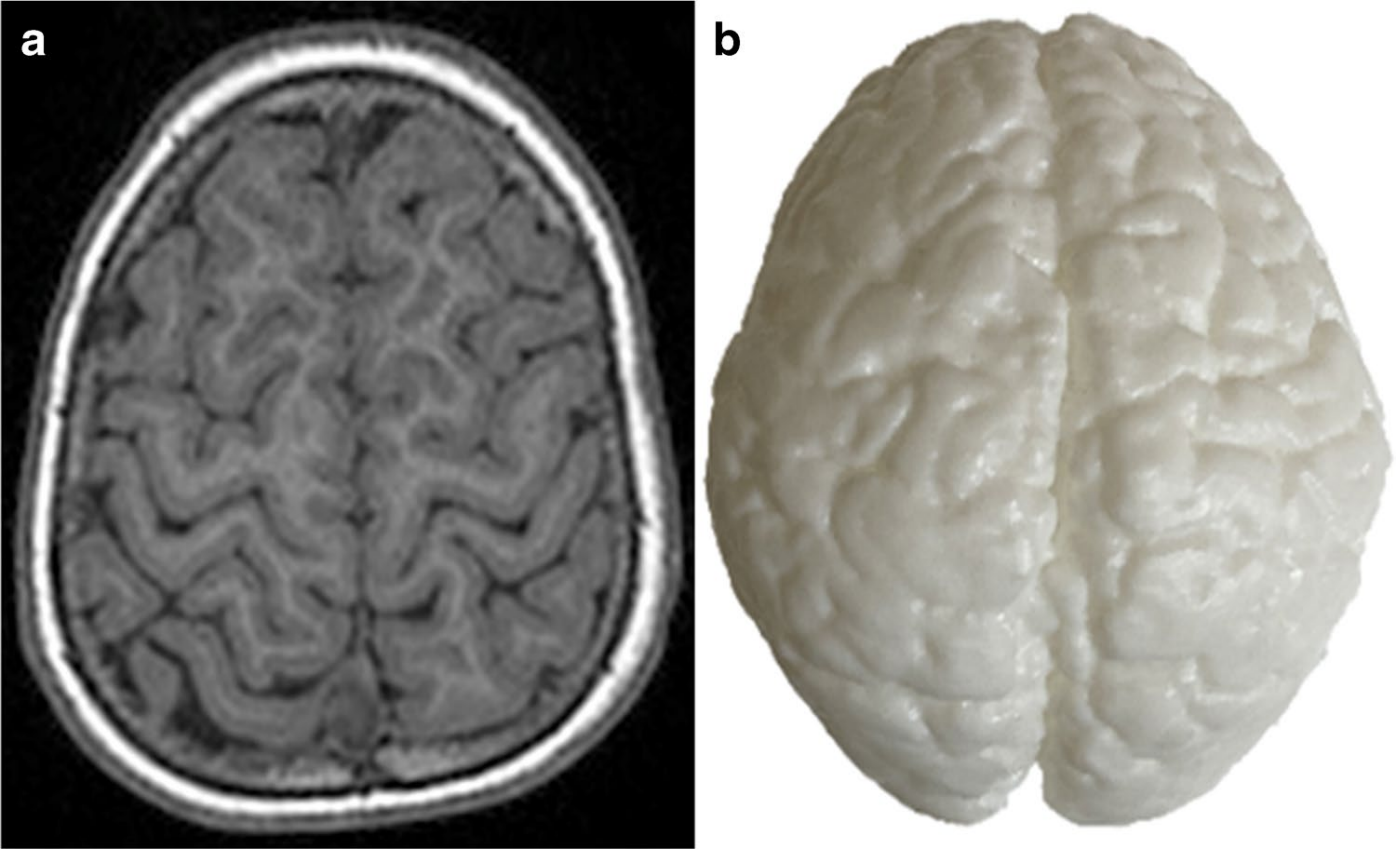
The normal cortex of the brain in a 5-year-8-month-old girl presenting with cerebral palsy. a Axial T1-weighted magnetic resonance image shows the cortical surface at the vertex. b A vertex view of the corresponding 3-dimensional printed model which accurately depicts the cortical surface
